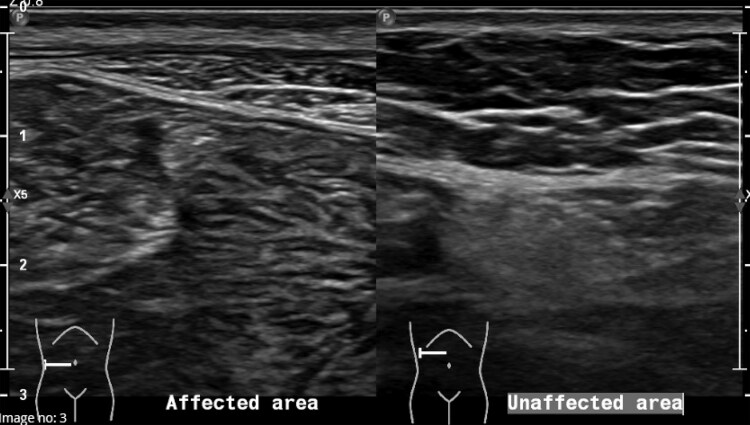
Ultrasound images. Ultrasound of the abdominal wall in the right lower quadrant shows that the affected lipoatrophic area has 3 mm of subcutaneous fat, whereas the unaffected adjacent region has 11 mm of subcutaneous fat.
